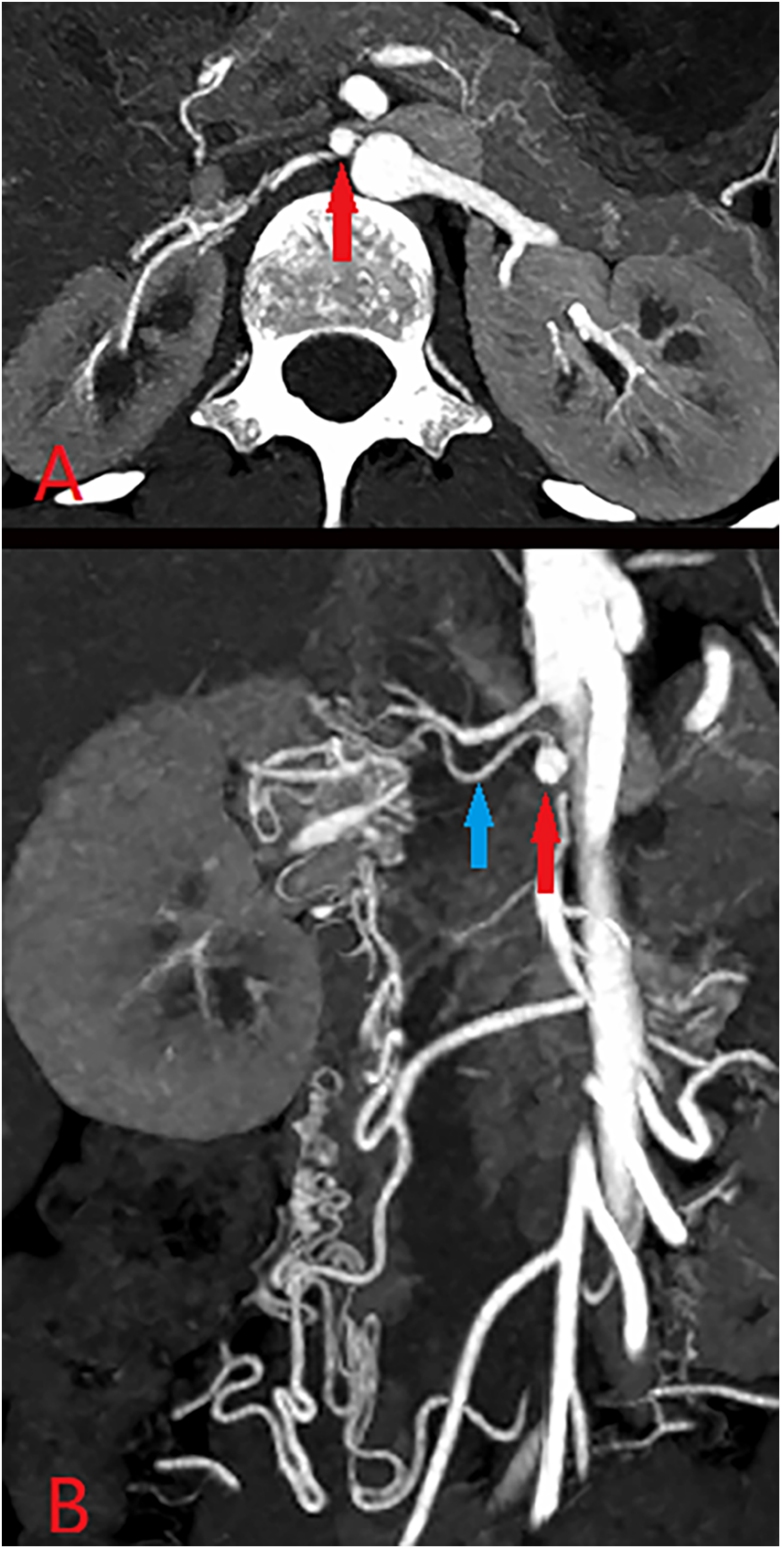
CTA (A,B) revealed that the aneurysm (approximately 0.5*0.5 cm, red arrow) was located proximal to the renal artery (blue), and several branch arteries formed the collateral circulation for the right kidney.
